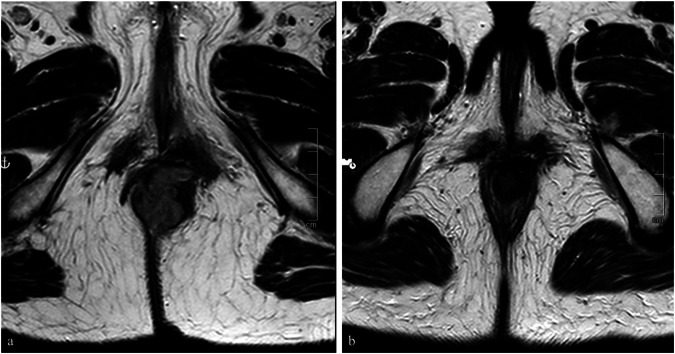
Turbo spin-echo T2-weighted paraxial images demonstrate no residual disease after combination CRT before (a) and after treatment (b): the patient was classified as a CR case
